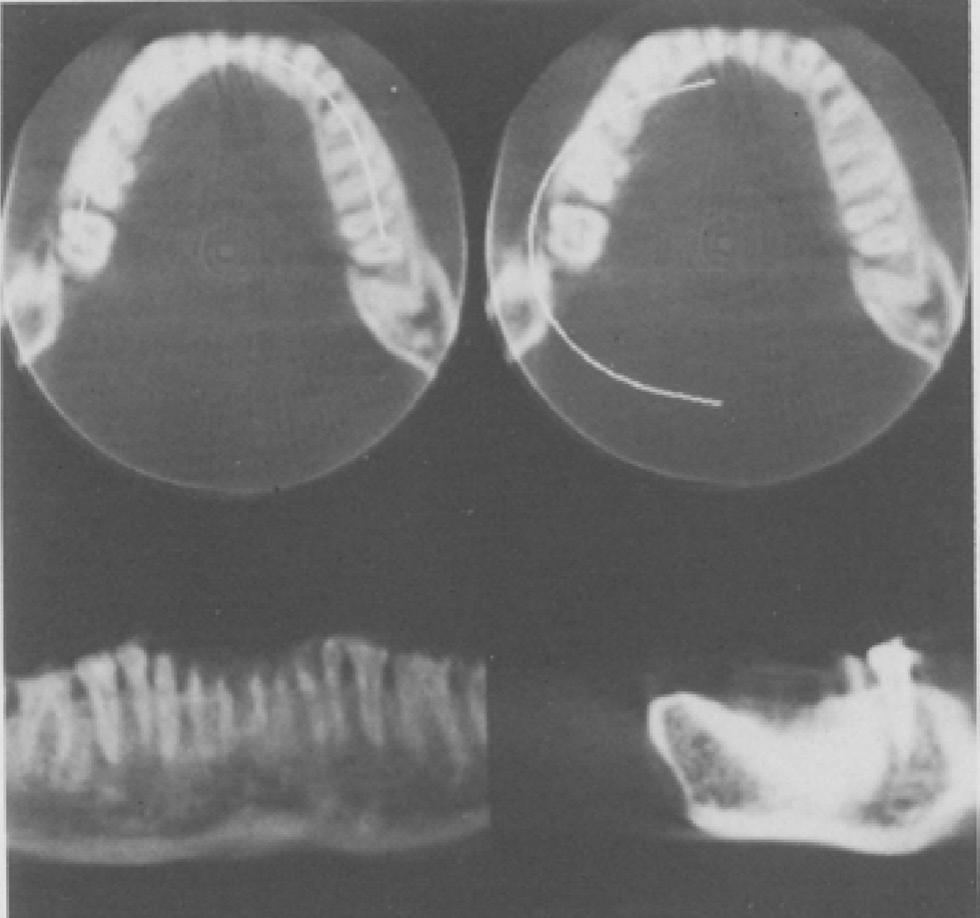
First cone beam CT images of jawbone.^7^
